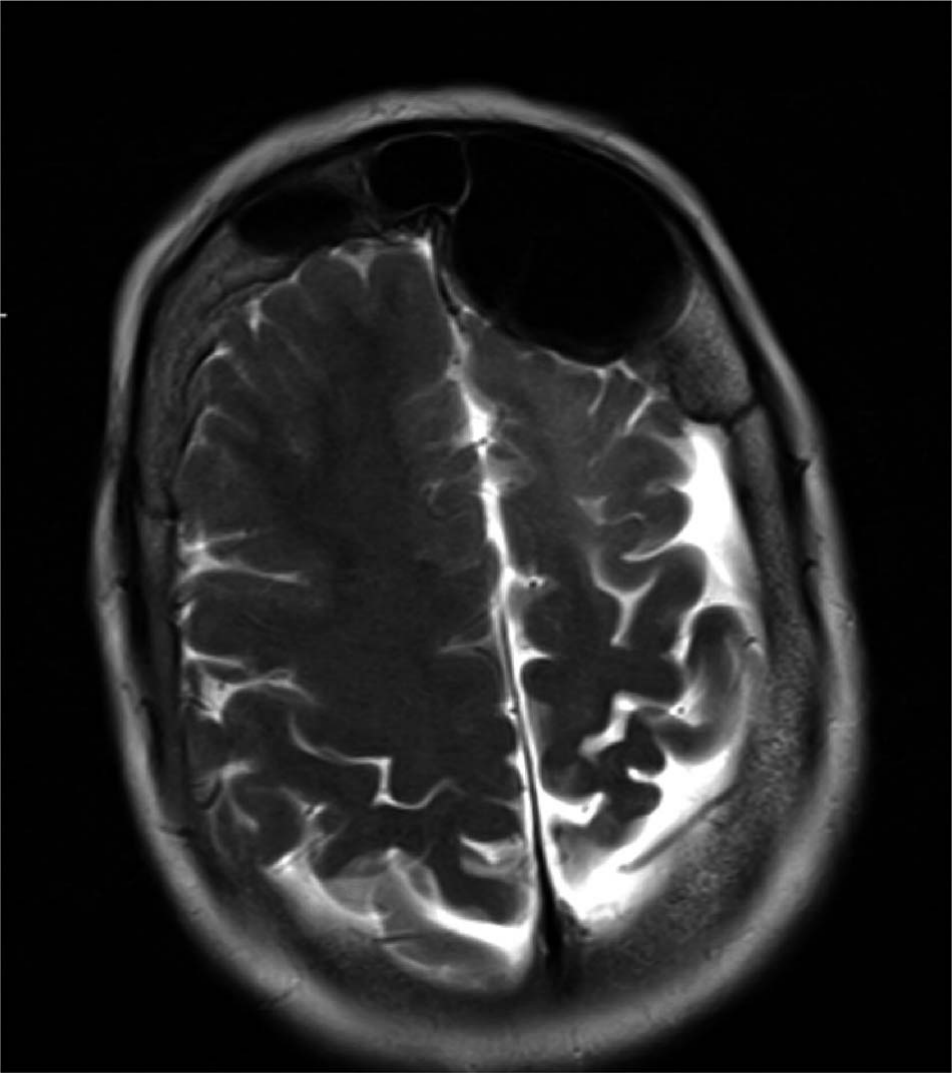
MRI showing enlarged left frontal sinus with mass effect on the frontal lobe.
